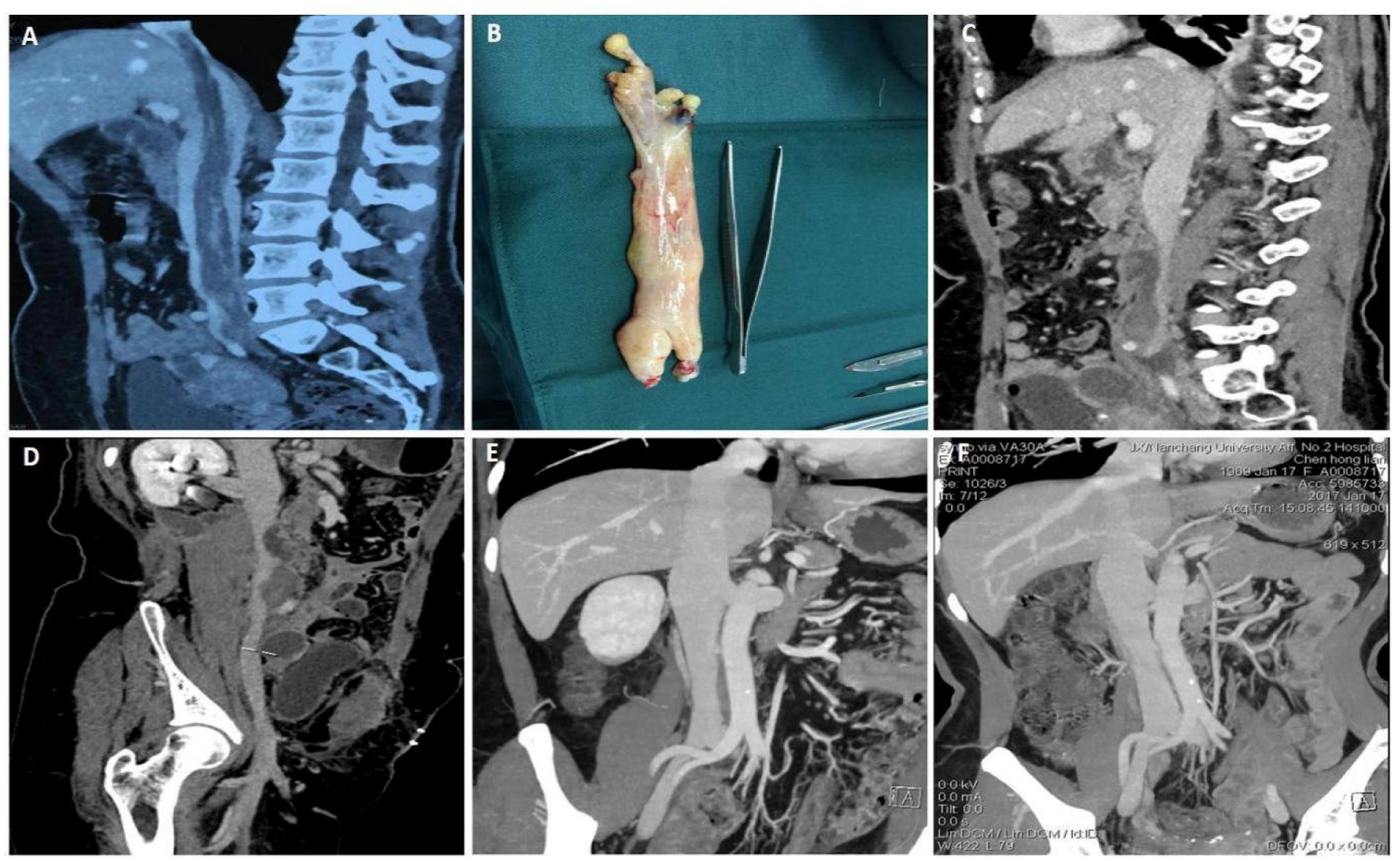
Data of case 1 before, during, and during follow-up. (A) Preoperative computed tomography venography (CTV) confirmed a mass that extended from the right internal iliac vein to the proximal inferior vena cava and extended into the right atrium. (B) An intact yellow mass was removed from the right atrium and proximal to the inferior vena cava. (C,D) Seven days after surgery, CTV confirmed that the mass was removed and the inferior vena cava was unobstructed. (E,F) Six-month follow-up CTV and 12-month follow-up CTV revealed no residual mass or recurrence and the prognosis was good.
